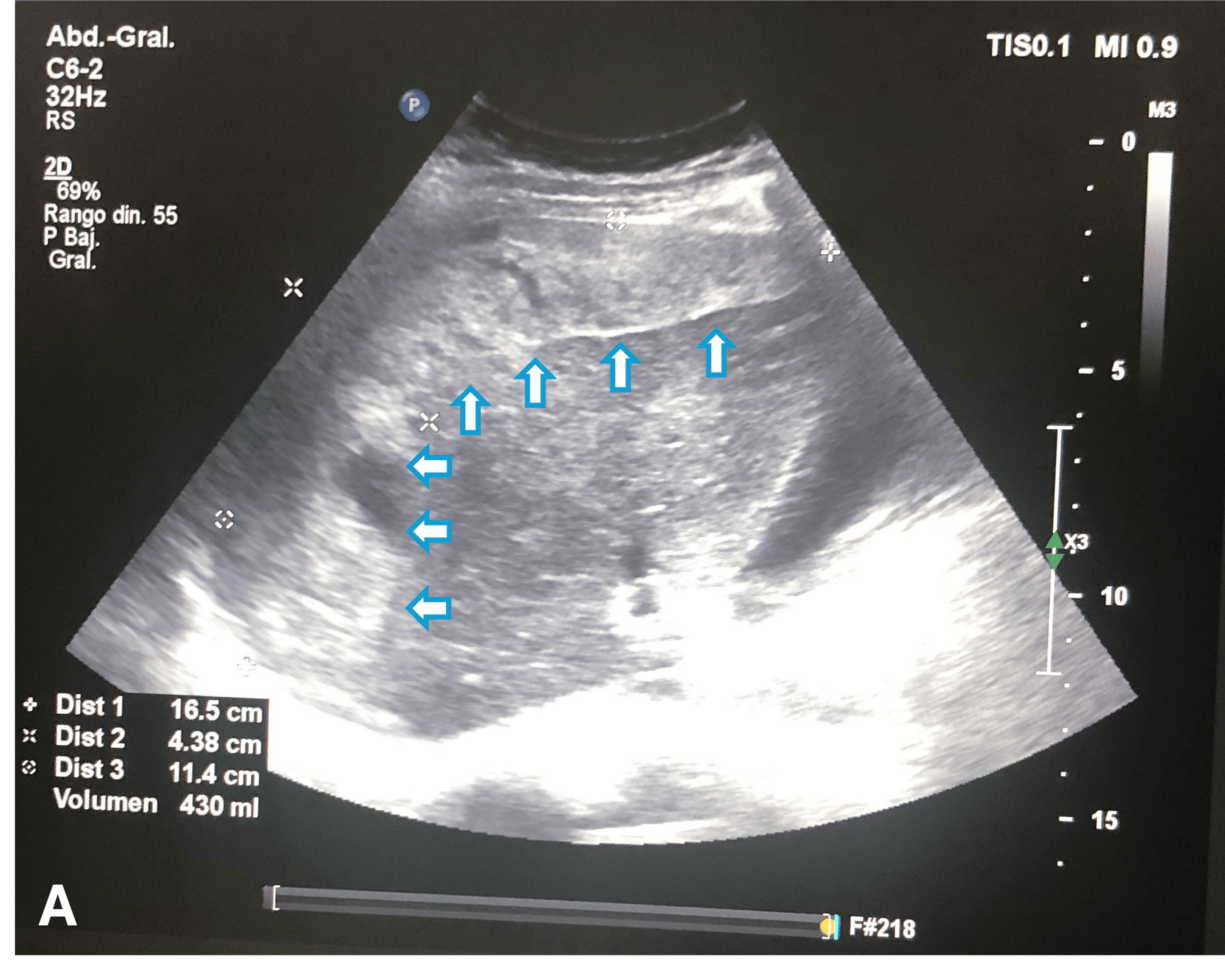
Abdominal ultrasound showing a subcapsular hepatic hematoma in the right lobe.
